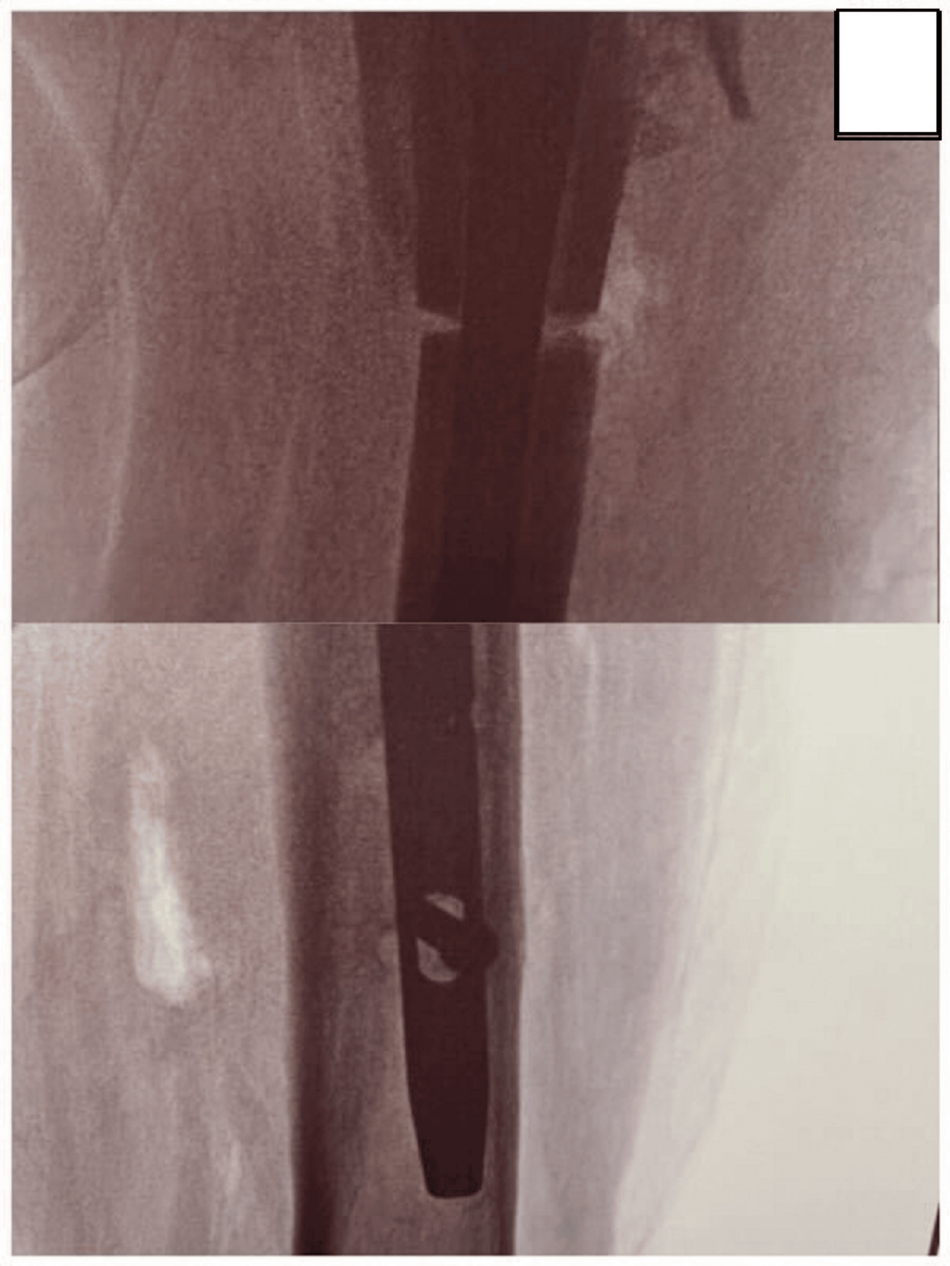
Intraoperative radiographs Intraoperative C-arm images from the second operation showing the femoral osteotomy (AP view, above) and the newly placed distal interlocking screw (lateral view, below).
